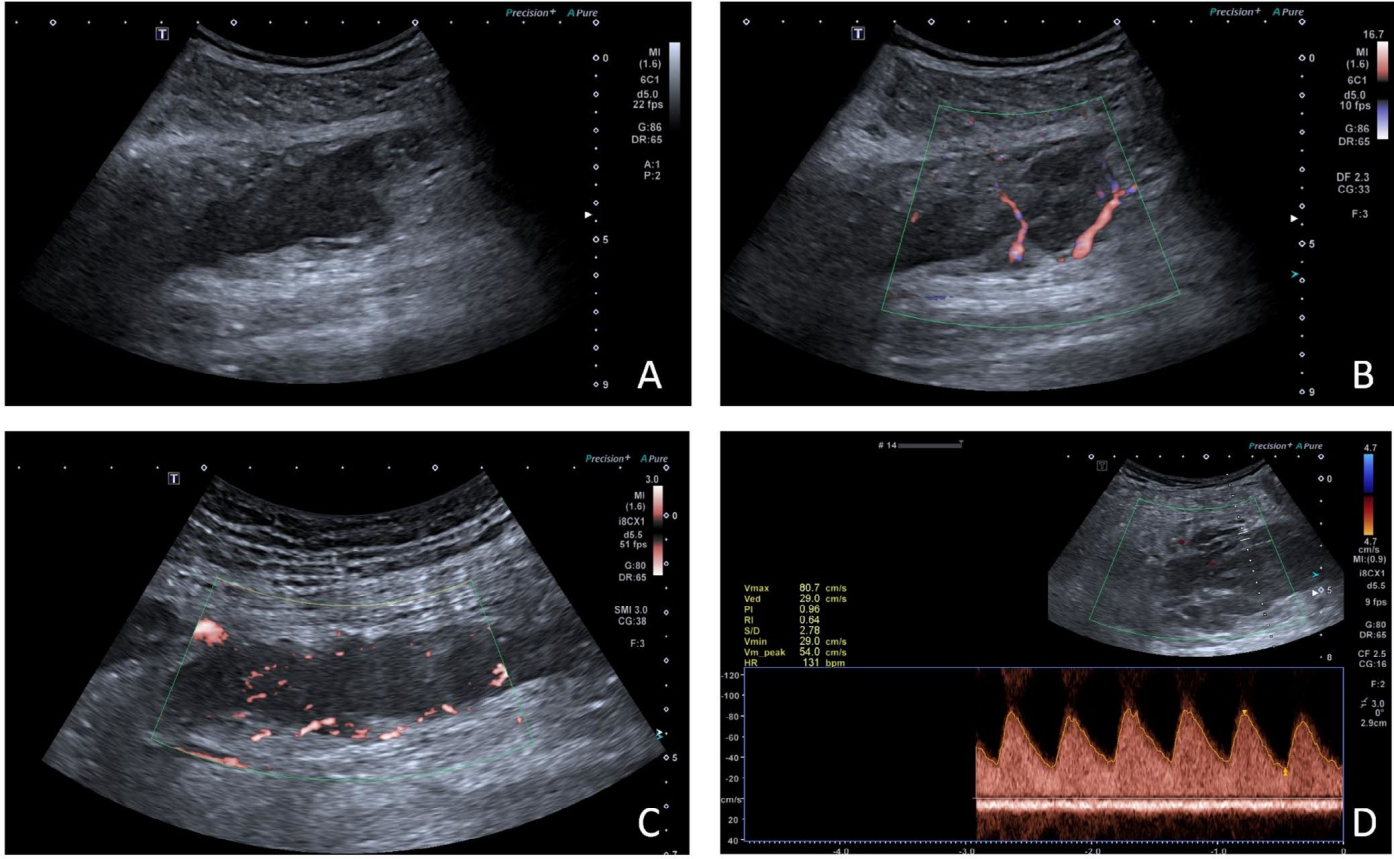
(A) Hypoechoic area within the calf muscles, with unclear borders and irregular shape. (B) CDFI shows strip‐like blood flow signals within the lesion. (C) SMI demonstrates blood flow signals within the lesion; (D) Pulsed Wave Doppler (PW) shows a high‐speed, low‐resistance blood flow spectrum within the lesion.
